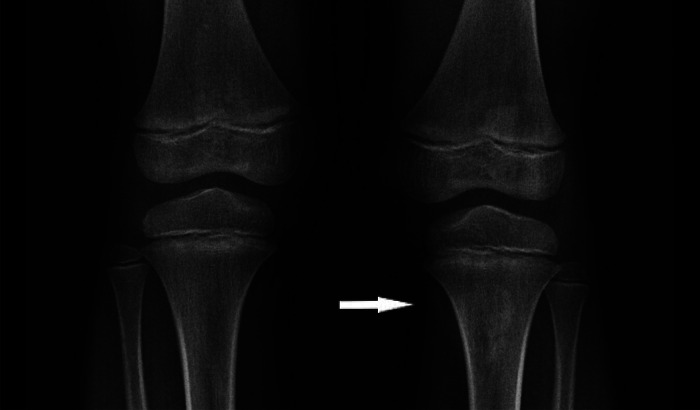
Antero-posterior view knee X-ray. Irregular opacity at the site of the anterior tibial tuberosity of the left knee (arrow) compared to the normal right knee.
